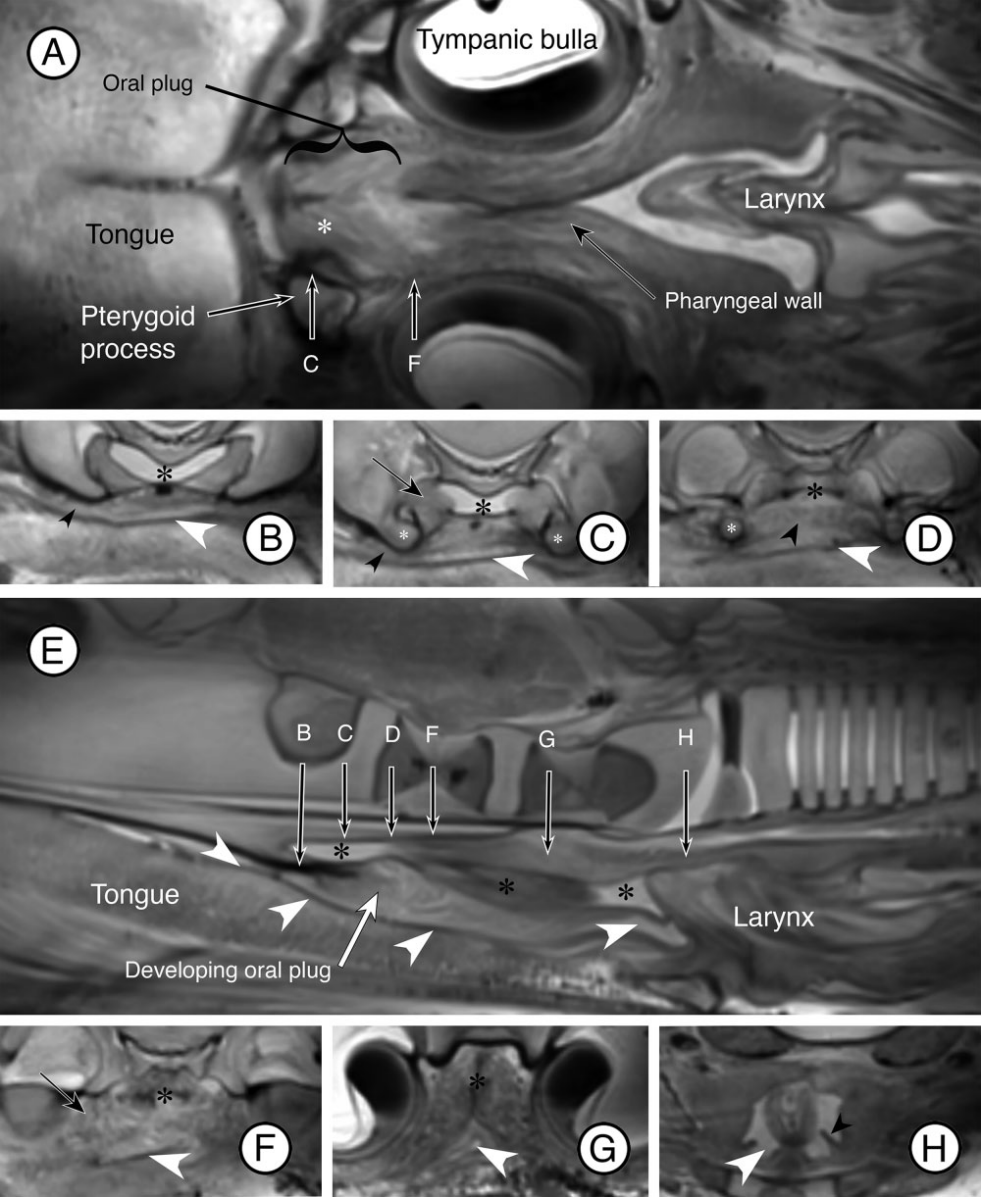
MRI scans that show developing muscles and mucosal folds of the soft palate in a fetal fin whale. Images in the dorsal and mid-median planes are shown in (A) and (E), respectively. Arrows with letters in these images are the approximate positions at which the corresponding images in the transverse plane (B–D; F–H) are taken. Dorsal is up in these transverse sections, and the black asterisks indicate the airway, and the white arrowheads indicate the “food” or “gut” pathway. In (B, C) the small black arrowheads indicate the developing palatoglossal fold and muscle. In (C, D) the small white asterisks indicate the developing pterygoid processes. In (C) the part of the developing levator veli palatini is indicated by the arrow, which is also visible near the asterisk in (A). In (D) the small black arrowhead indicates the developing tensor veli palatini muscle between the two pterygoid processes. In (F), the arrow points to another part of the developing levator veli palatini muscle, which is also indicated by the white asterisk in (A). In (H) the small black arrowhead points to one of the palatopharyngeal folds.
